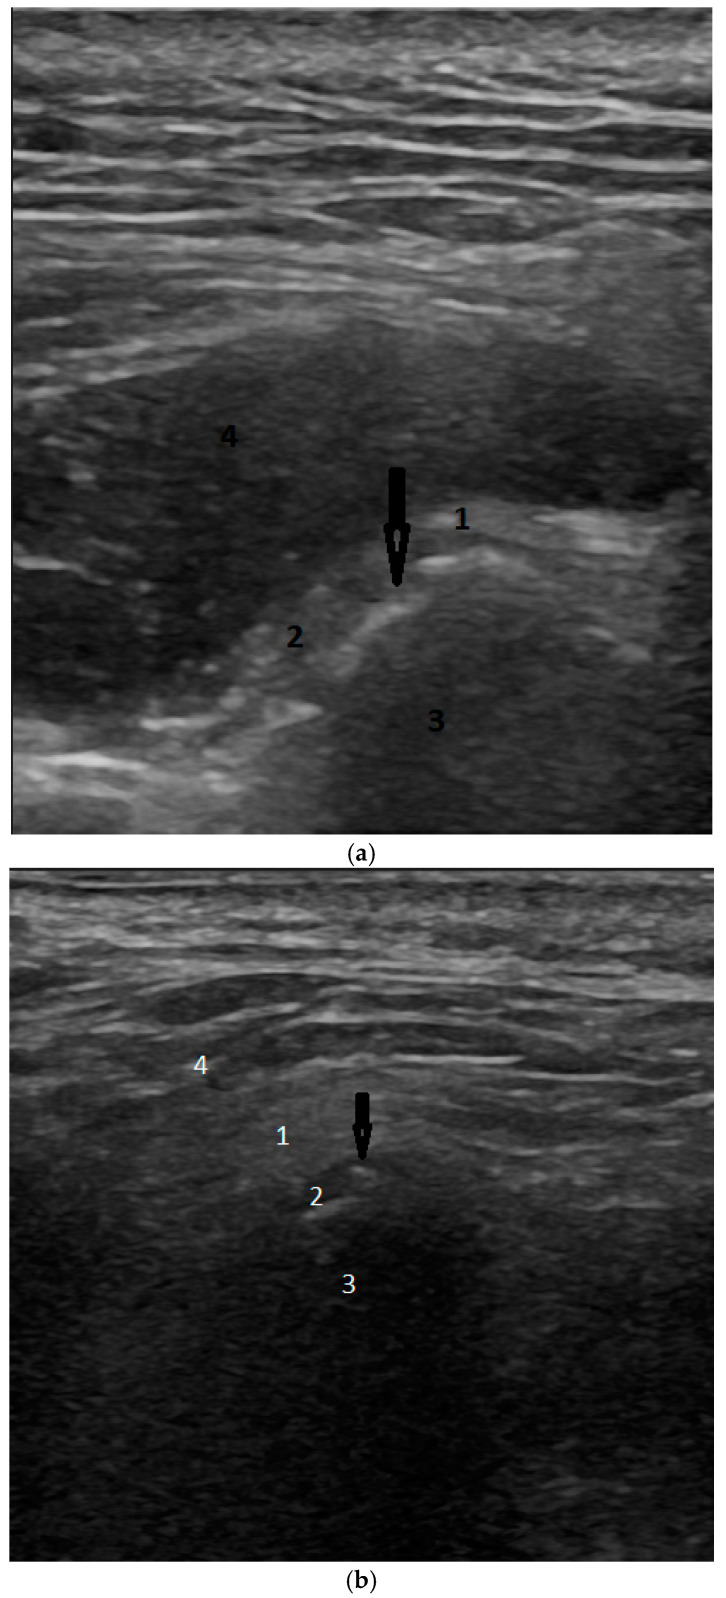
(a) Ultrasonography (sagittal view). Black arrow is pointing to erosion. Anatomical structures: 1. Glenoid Fossa, 2. Disc, 3. Condyle, 4. Masseter muscle; (b) Ultrasonography (axial view). Black arrow shows osteophyte. Anatomical structures: 1. Glenoid Fossa, 2. Disc, 3. Condyle, 4. Masseter muscle.
